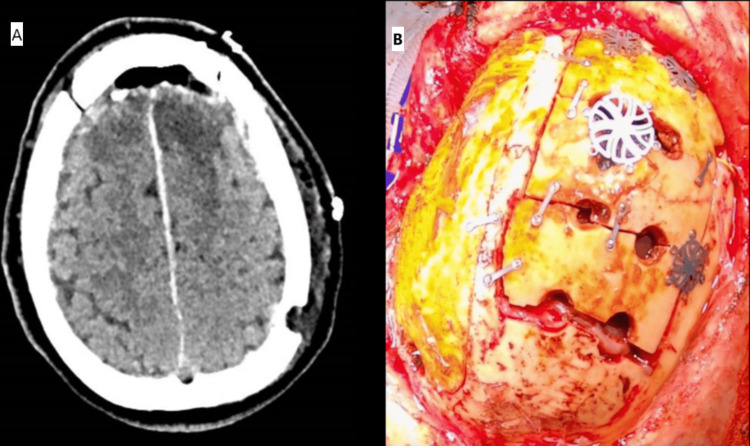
Postoperative plain CT head (A) of case 1 showing satisfactory appearance and intraoperative photograph (B), revealing midline island-like craniotomies over the superior sagittal sinus CT: computed tomography
